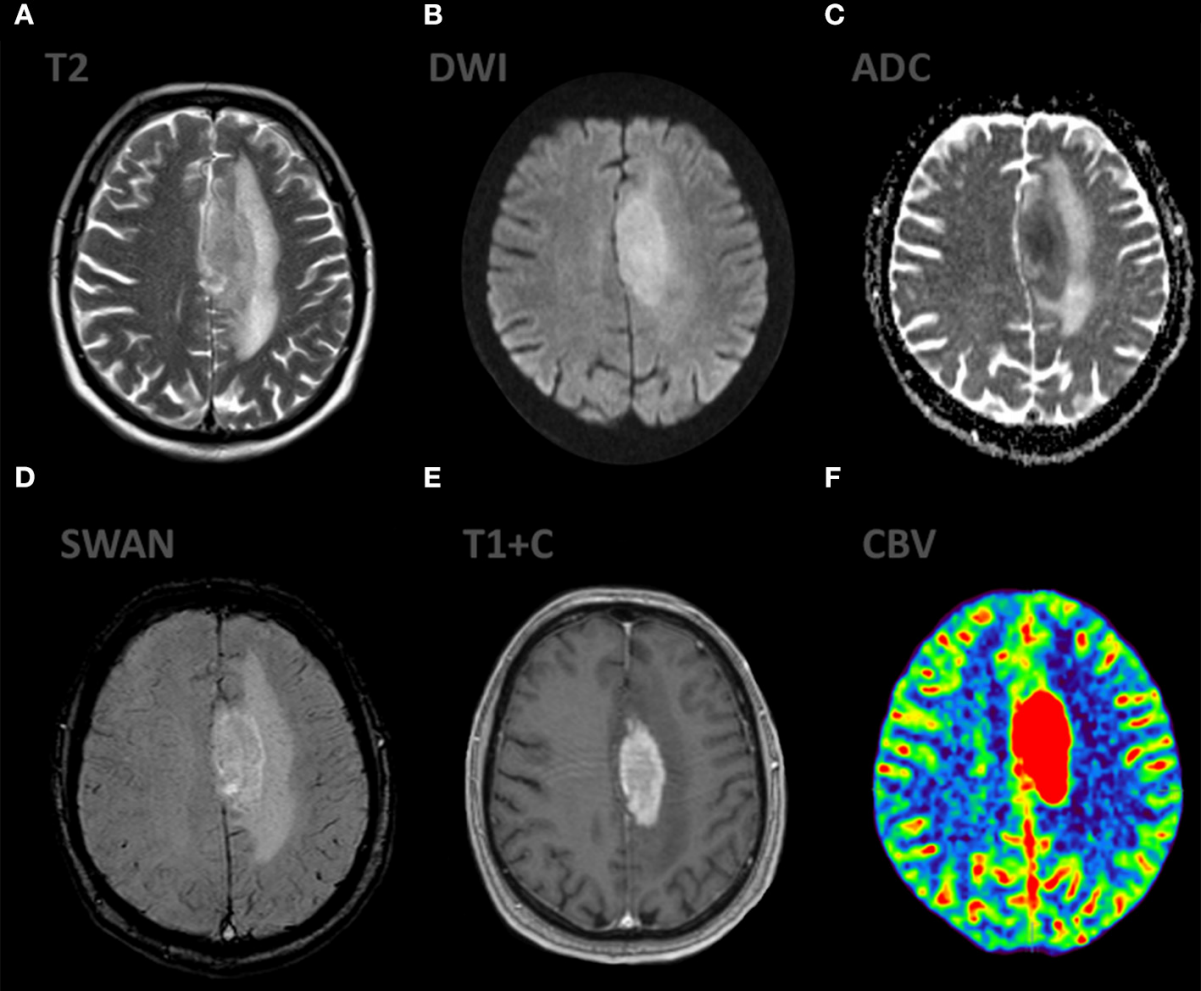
Patient V., male 61 y.o. presented with acute right sided weakness. Axial Brain MRI and CT-perfusion. MRI ax: (A) T2WI; 6, (B) DWI, (C) ADC, (D) SWAN, (E) T1 + CE. CT ax: (F) CT-perfusion (CBV map). There is a well circumscribed mass in the left frontal region with surrounding vasogenic edema on T2WI. The mass has low SI on ADC map indicating high cellular density. The contrast enhancement is bright and uniform. CT-perfusion map shows increased rCBV level.
